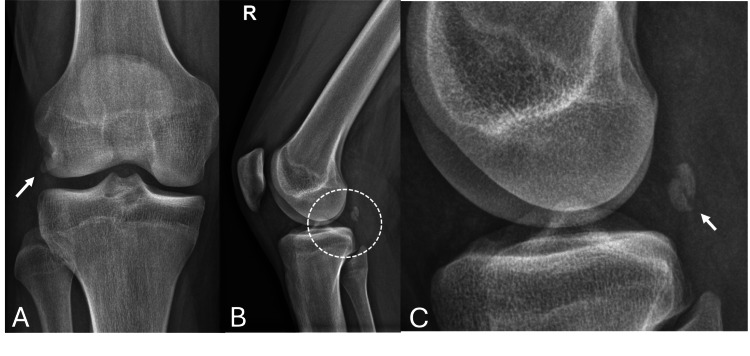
Right knee X-ray. (A) Anterioposterior view showing fabella overlapped by lateral femoral condyle (white arrow); (B) lateral view and (C) magnified image showing a fractured right fabella with minimally displaced fragments (white arrow)
